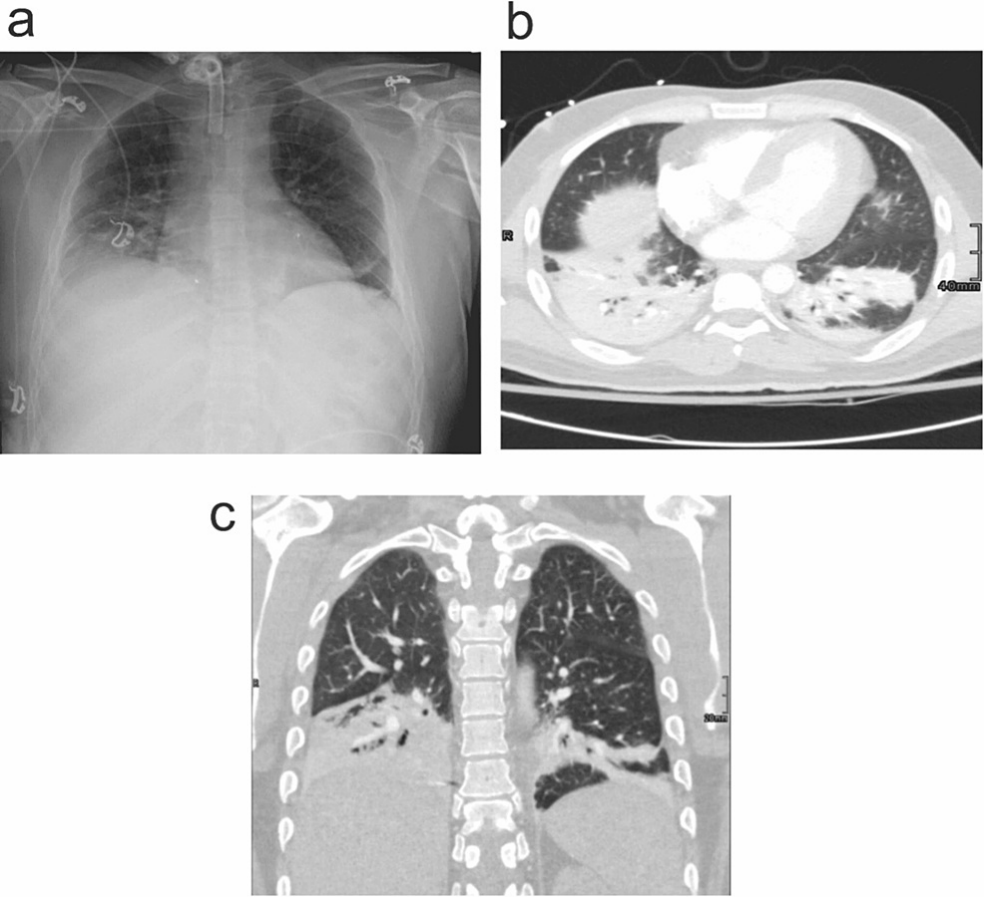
Radiologic findings in patients with nervous system injuries and developing pneumonia a. The radiograph demonstrates right basilar pleuroparenchymal and left basilar linear airspace opacities. No large pleural effusion or pneumothorax is identified. b. Axial view of the CT chest with contrast which was performed the next day for evaluation of a possible pulmonary embolism shows bibasilar consolidative opacities with air bronchograms. No segmental or sub-segmental pulmonary embolism was identified. c. Coronal view of the same patient in b.
